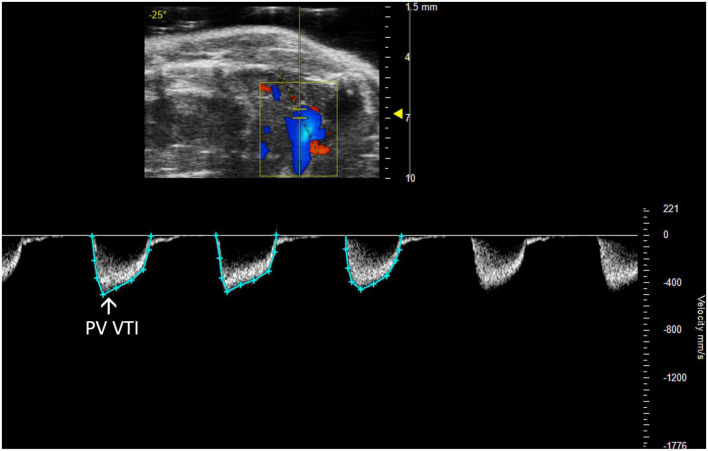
The pulmonary valve velocity time integral (PV VTI), a measure of blood flow through the pulmonary valve, is calculated by measuring the area under the curve in pulsed wave (PW) Doppler mode of the parasternal long axis view (PLAX).
